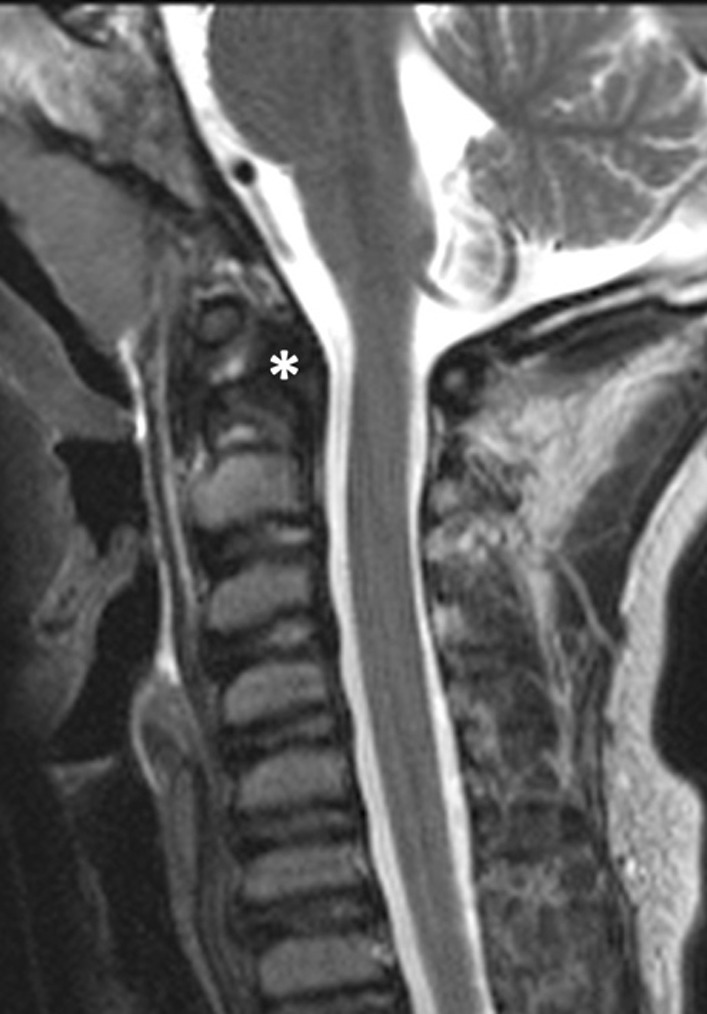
Sagittal T2-weighted TSE MRI of the cervical spine of Patient 1 at 10 years of age shows dysplastic odontoid process of C2, with irregular sclerosis and posterior migration of the tip of the dens (asterisk)
